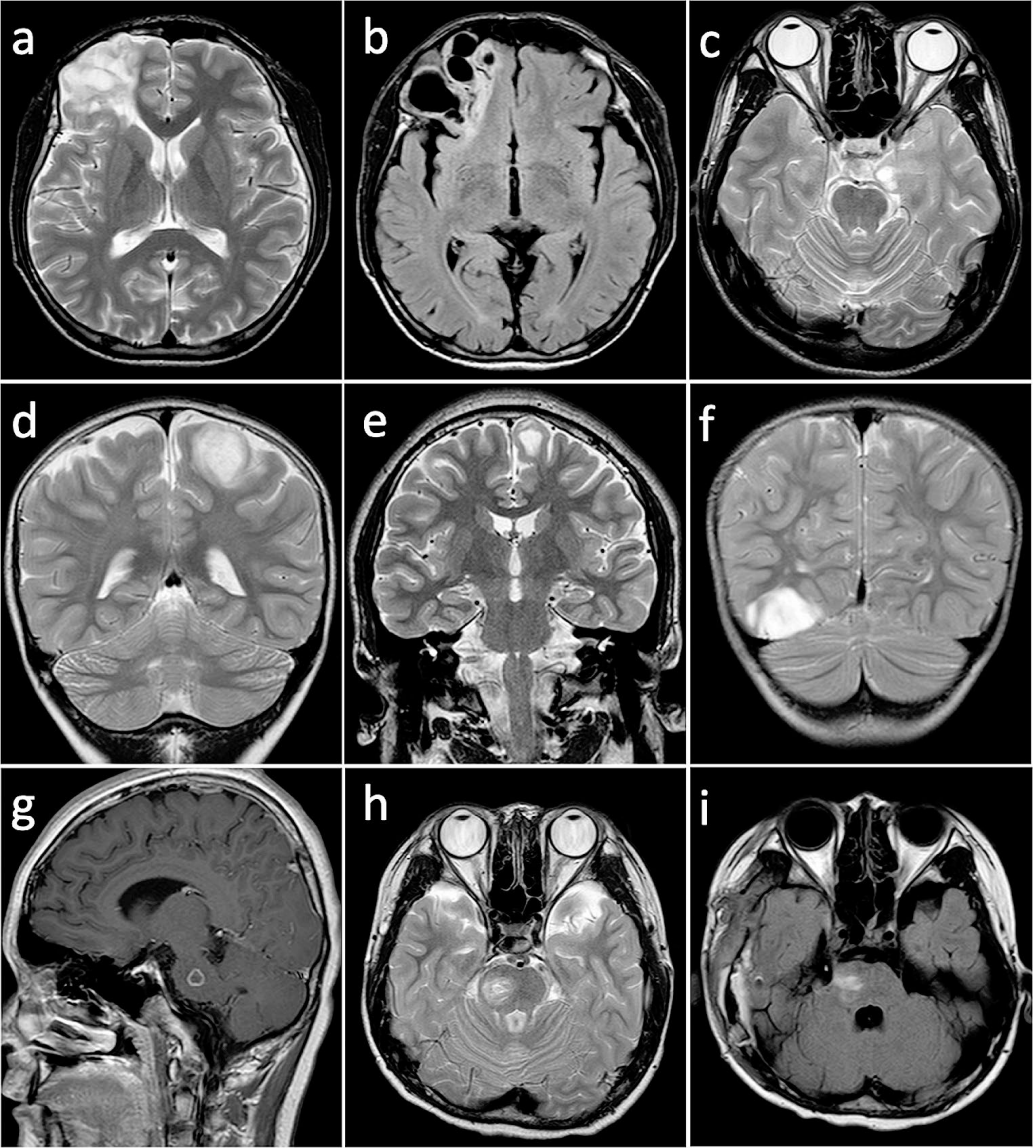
a, b. Case 1, MRI showed a polycystic lesion with high T2-weight signal and rim-like high FLAIR signal in the right frontal lobe; c. Case 2, T2-weighted image showed high signal in the left mesial temporal lobe; d, e,f. Case 9,10,11, stalk-like signs and brain parenchyma atrophy can be observed; g, h,i. Case 14, lesion was observed in the right side of pons
